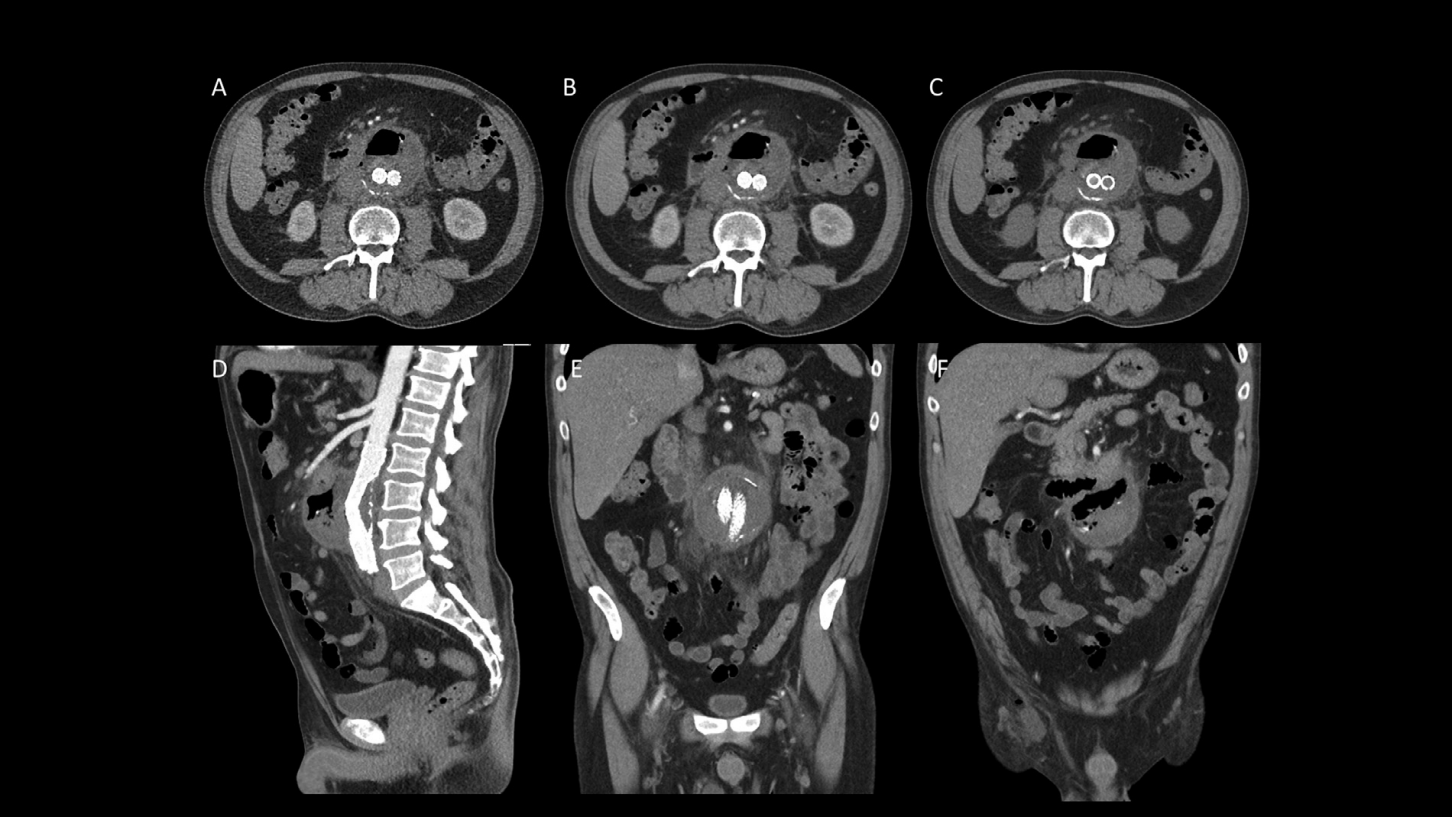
Five days following the procedure patient reported bright red blood per rectum with stable vitals and hematocrit. CT angiogram of the abdomen and pelvis was performed. Arterial phase (A, D and E), delayed phase (B) and unenhanced study (C and F). It showed normal positioning of aortobiiliac stent graft without evidence of endoleak. Stable size of the excluded aneurysmal sac. There was interval worsening of ectopic gas within the excluded aneurysmal sac. Findings compatible with known aortoenteric fistula.
